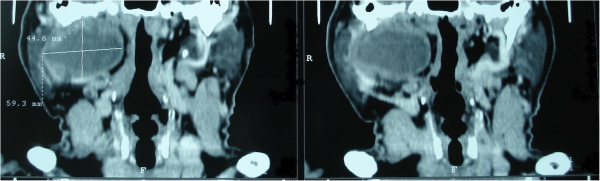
Computed tomography scan (coronal cuts): Homogenous and well defined cystic mass involving of the right parotid gland and measuring 44.6 mm × 59.3 mm.
